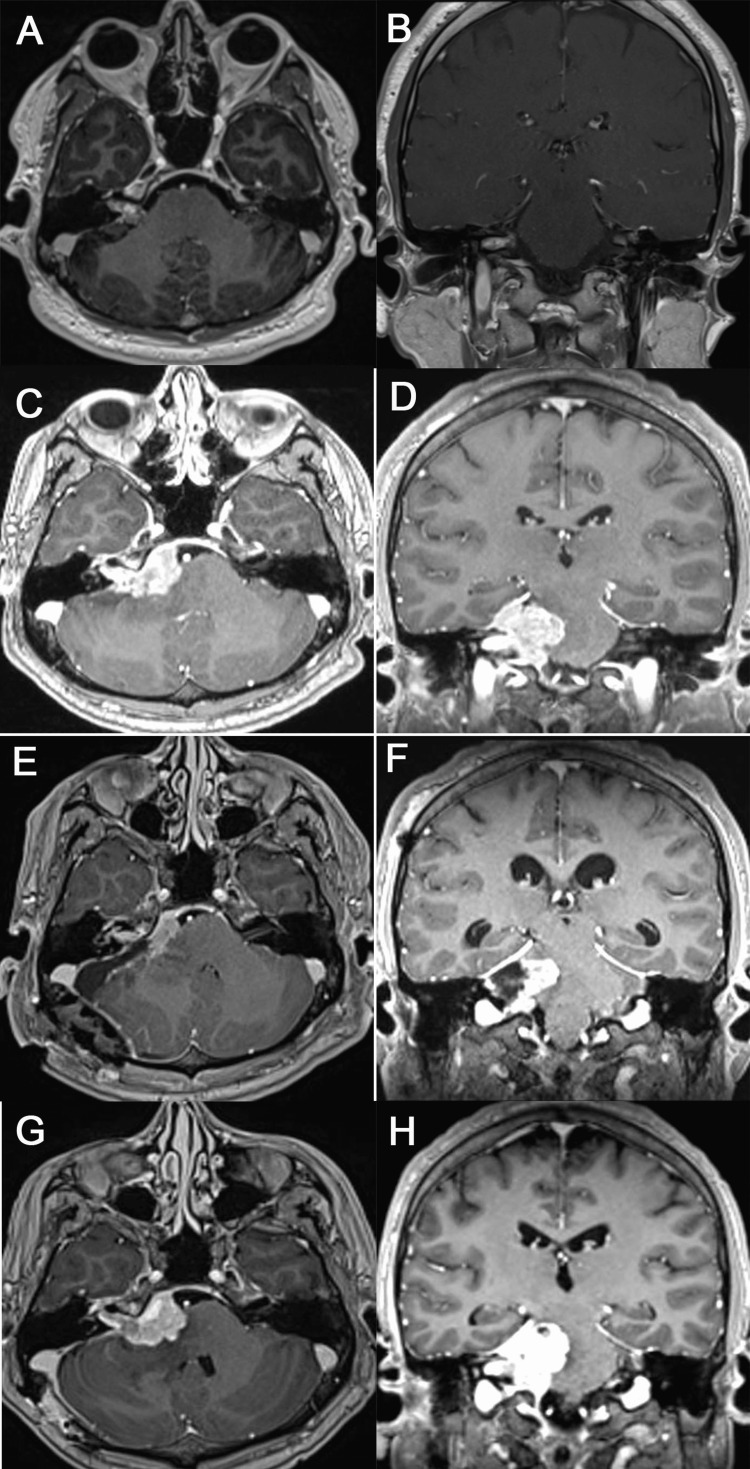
Preoperative and follow-up magnetic resonance imaging Contrast-enhanced T1-weighted axial (A) and coronal (B) demonstrates a mass in the right internal acoustic canal. Six months after radiosurgery treatment contrast-enhanced T1-weighted axial (C) and coronal (D) reveals tumor growth with compression of the brain stem. After the right retromastoid craniectomy approach, contrast-enhanced T1-weighted axial (E) and coronal (F) demonstrated residual tumor. Four cycles after chemotherapy, contrast-enhanced T1-weighted axial (G) and coronal (H) showed tumor progression.
